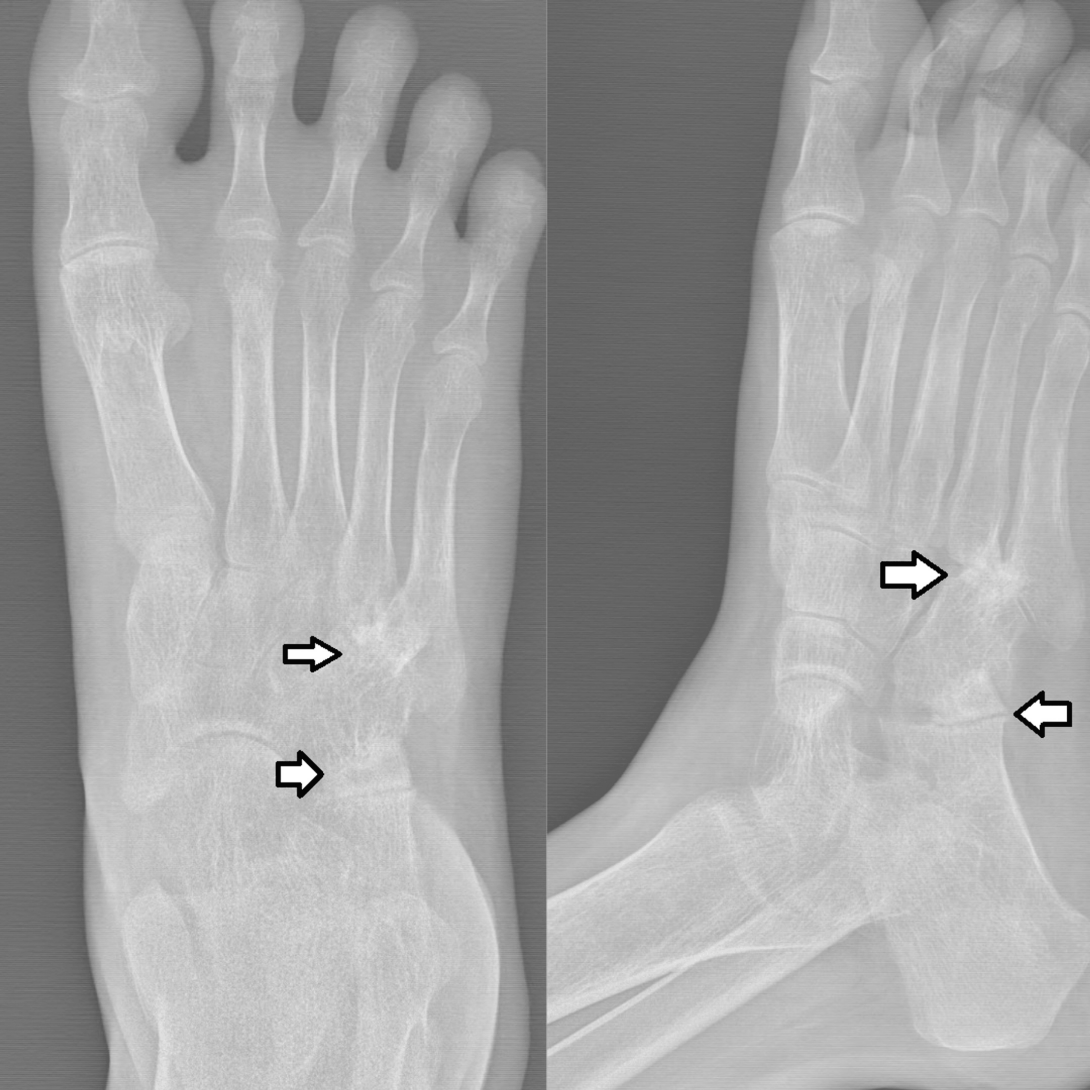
Conventional X-ray of the right foot in anteroposterior and oblique views taken six months post procedure, with arrows indicating bone graft consolidation.
